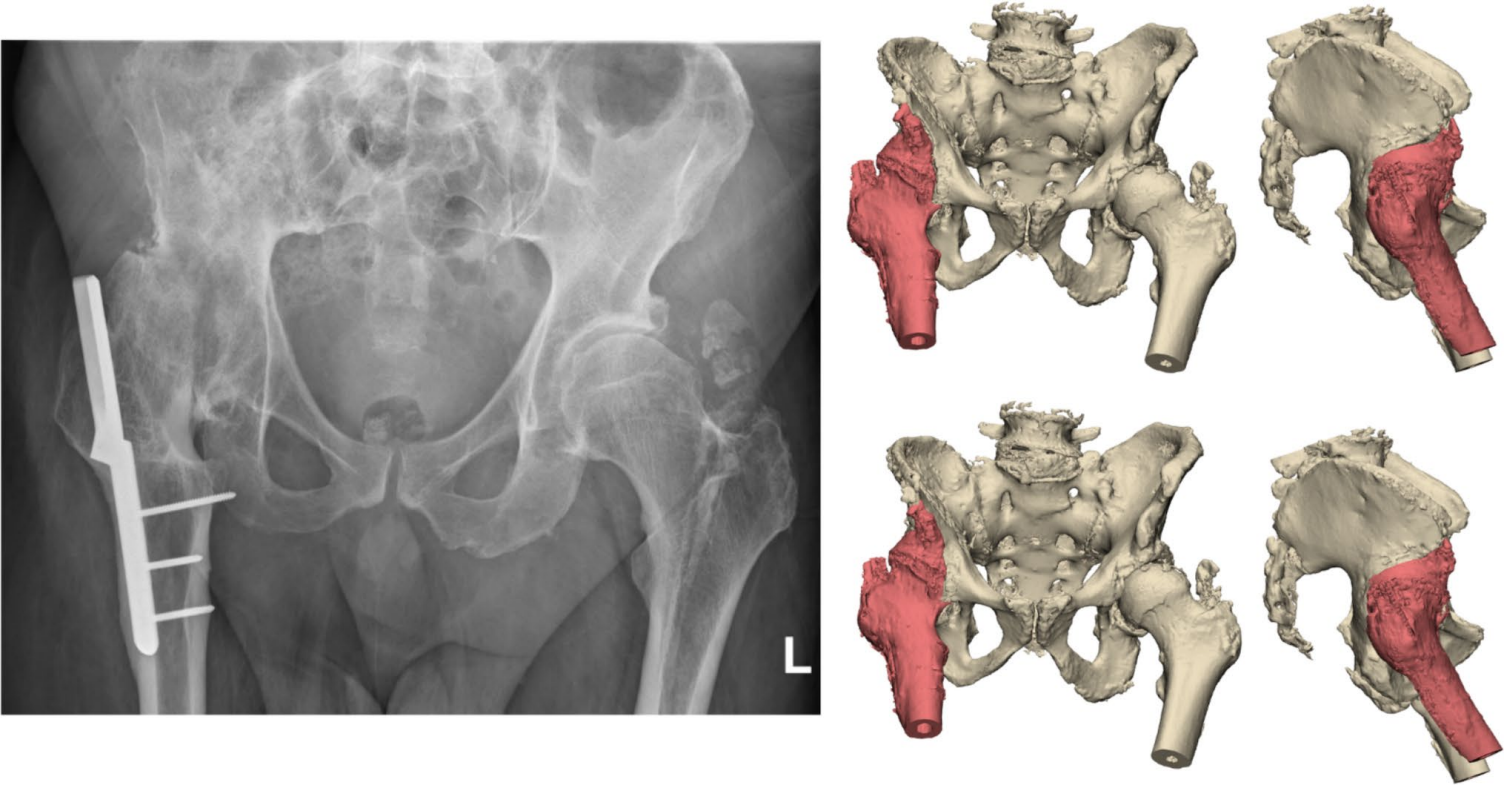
Case 2. Preoperative X-ray and 3D reconstruction and reduction of the fracture of the right hip arthrodesis
